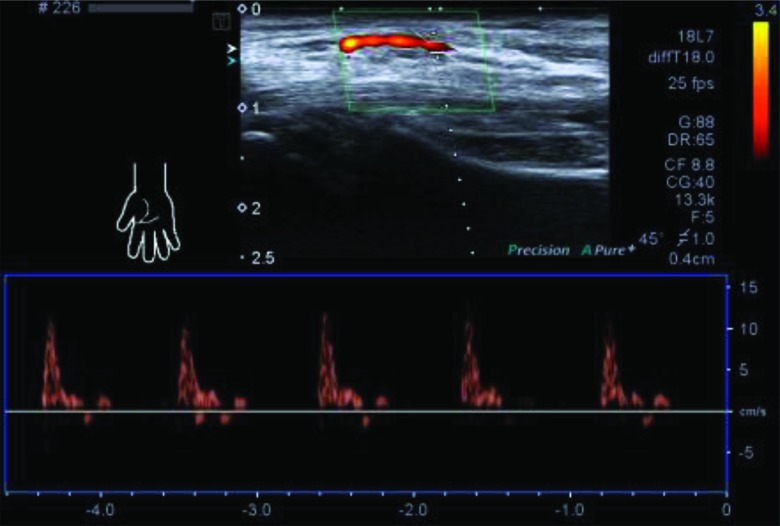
Spectral Doppler waveform of a PMA proximal to the carpal tunnel, which demonstrate peripheral arterial flow. The artery can be visualized in the middle of the two branches of a bifid median nerve.
